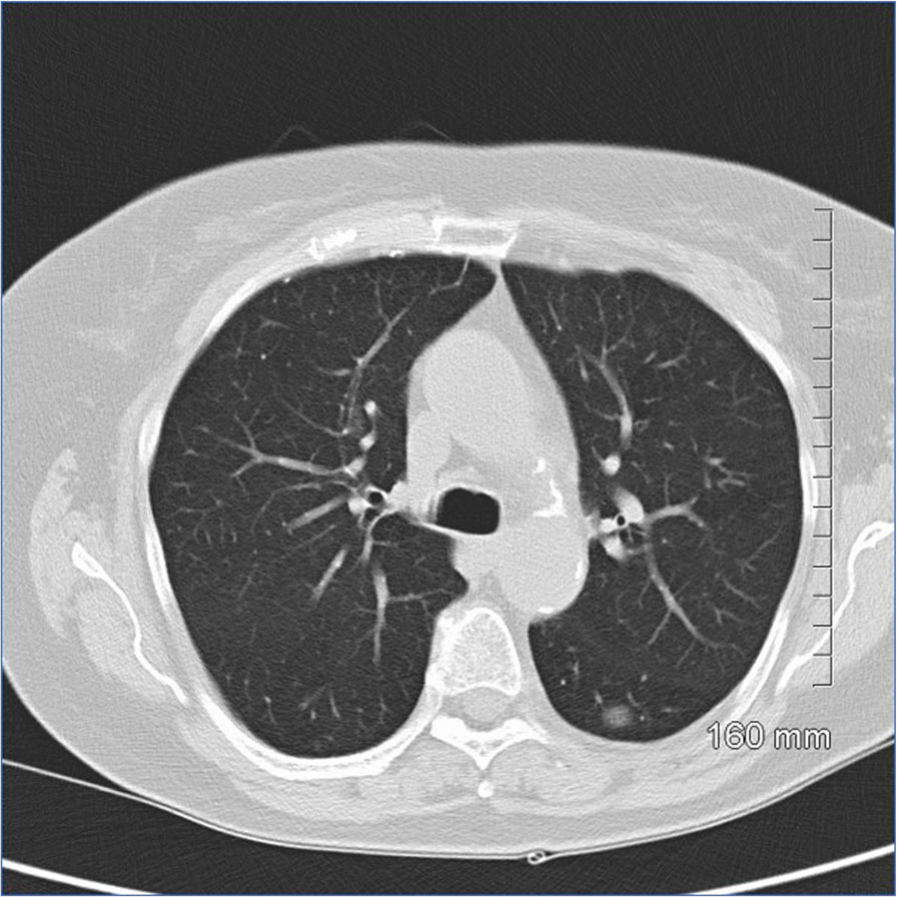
AIS on CT. A 10-mm pure ground glass nodule in the left lower lobe. Stable in size for 3 years prior to biopsy
